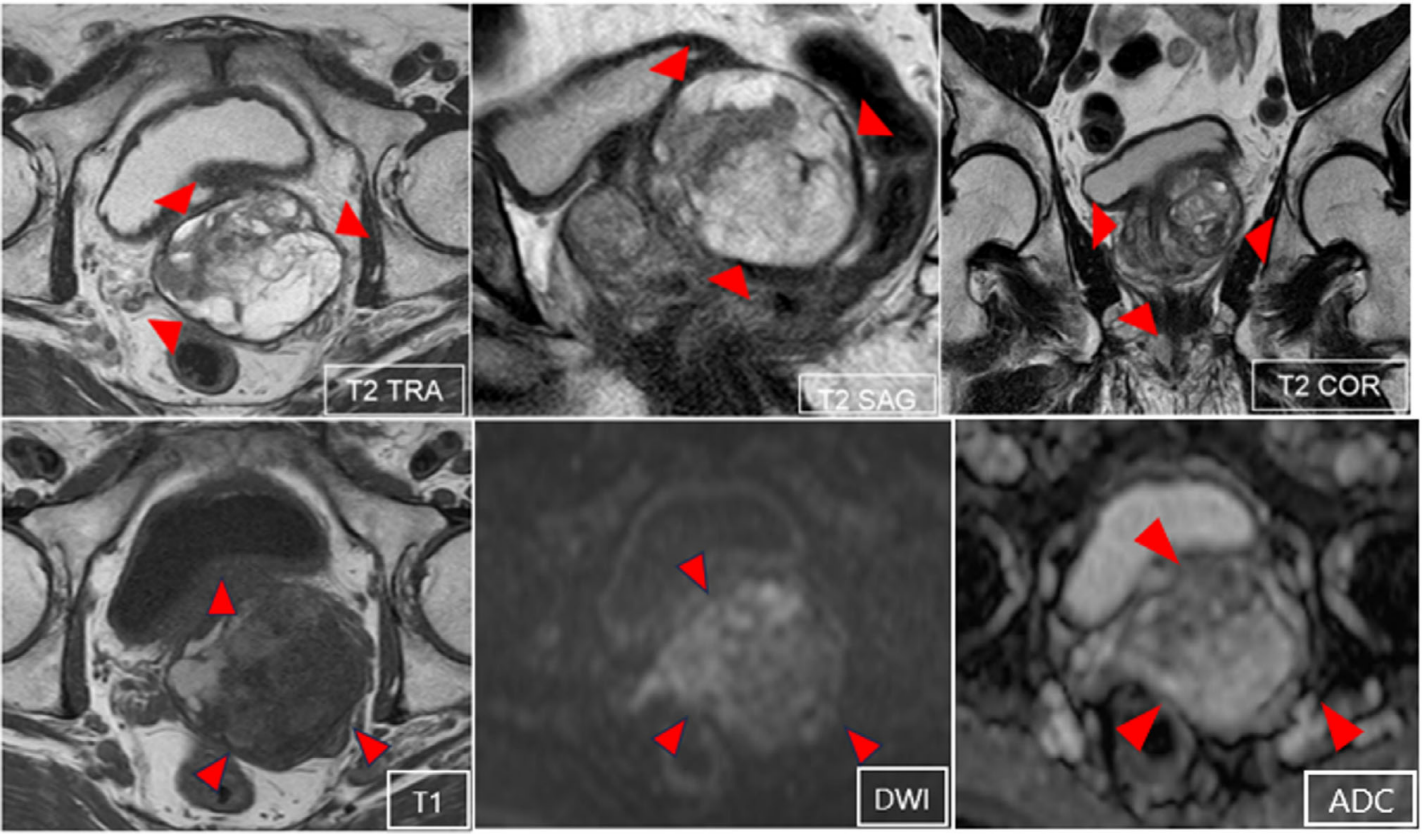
MRI revealed a 6.5 cm large multilocular mass with hemorrhage at the base of the left lobe of the prostate. ADC, Appearant diffusion co‐efficient; COR, coronal; DWI, Diffusion‐weighted imaging; RA, transverse; SAG, sagittal.
